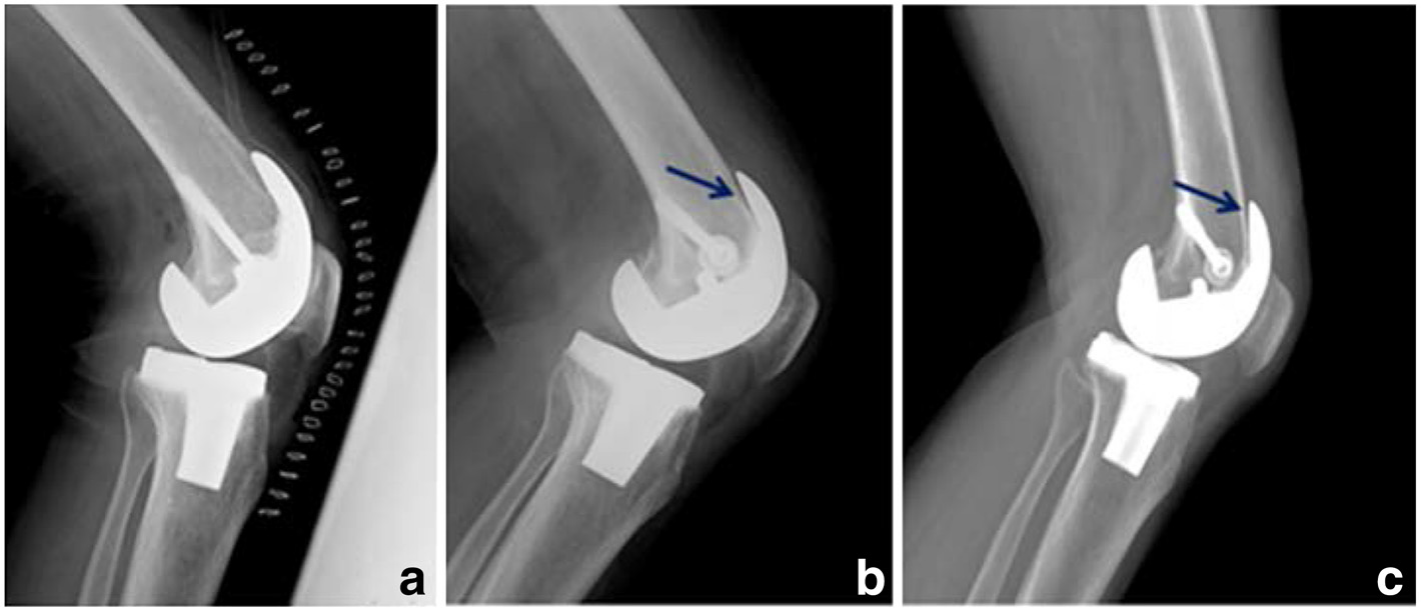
Left lateral knee radiograph showing a radiolucent line at the anterior surface of femoral component (*arrow*). a Immediately after surgery. b Postoperative 3 years. c Postoperative 5 years
